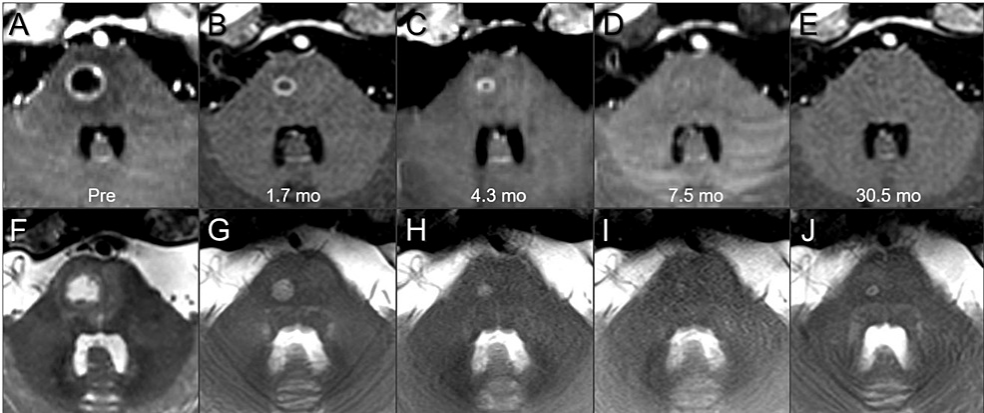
Serial magnetic resonance images for brain metastasis in the pons. The images show (A-E) axial CE-T1-WI; axial T2-WI (F-J); before fSRS (pre) (A, F); at 1.7 months (mo) after fSRS (B, G); at 4.3 months (C, H); at 7.5 months (D, I); and at 30.5 months (E, J). These serial image datasets were co-registered and fused on MIM Maestro^TM^ (Cleveland, OH: MIM Software). CE: contrast-enhanced; T1-WI: T1-weighted image; T2-WI: T2-weighted image
